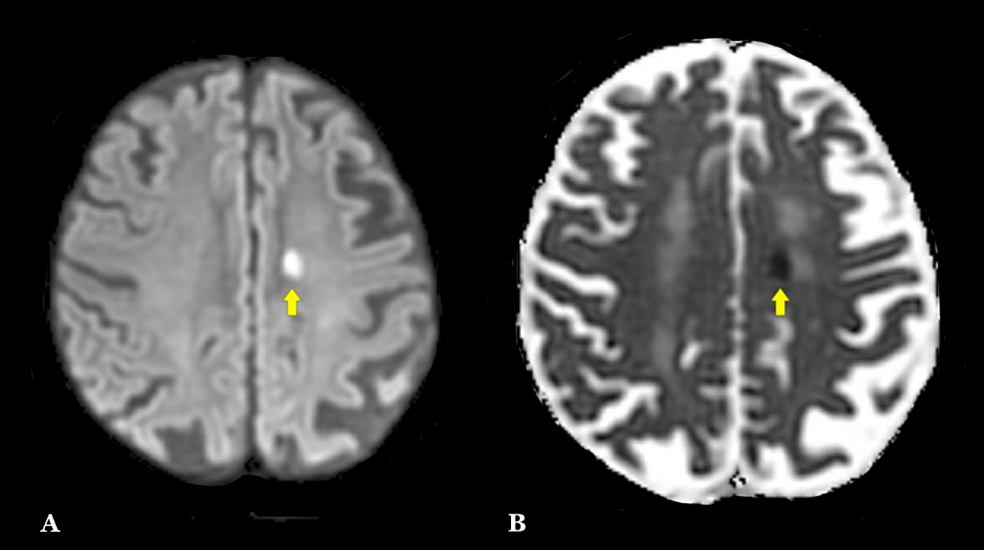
Patient’s magnetic resonance imaging (MRI) sequences The diffusion-weighted imaging (DWI) sequence in Panel A shows subcortical restricted diffusion along the left-sided periventricular region of the parietal lobe. Panel B shows the apparent diffusion coefficient (ADC) correlate of the same region signifying cytogenic edema due to acute/subacute ischemic infarction.
